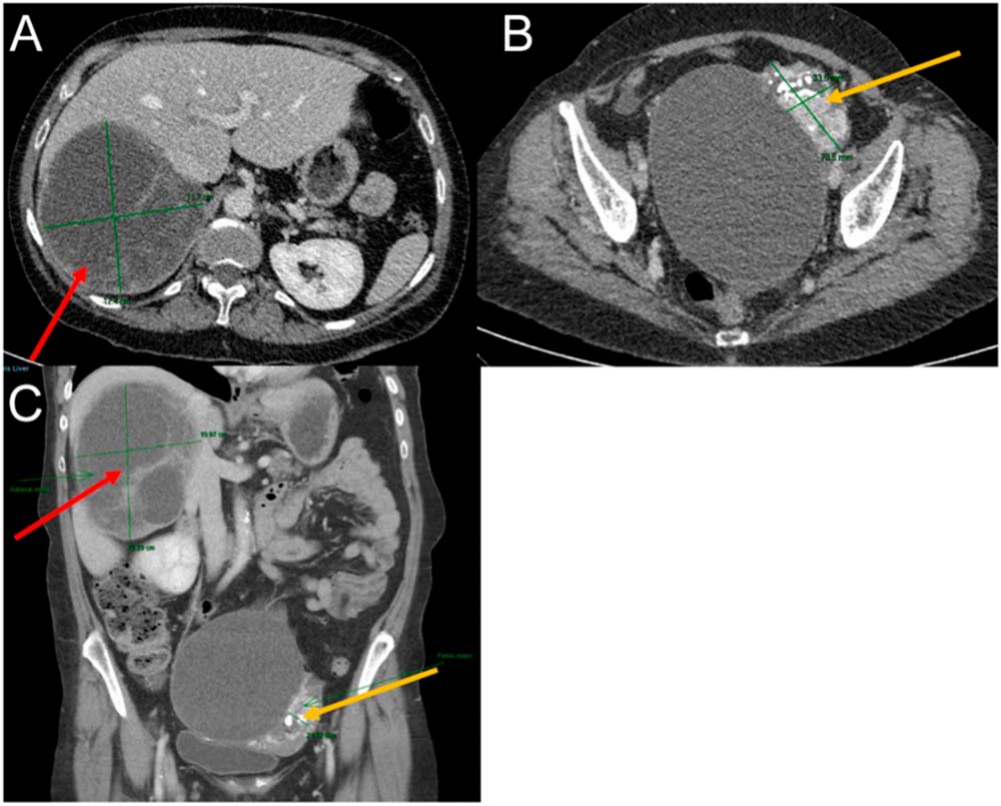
CT abdomen/pelvis in portal venous phase: (A) axial view of complex 15 cm right adrenal mass (red arrow), (B) axial view of heterogeneous 7 cm pelvic mass (yellow arrow), (C) coronal view of both masses (right adrenal mass (red arrow) and pelvic mass (yellow arrow)).
